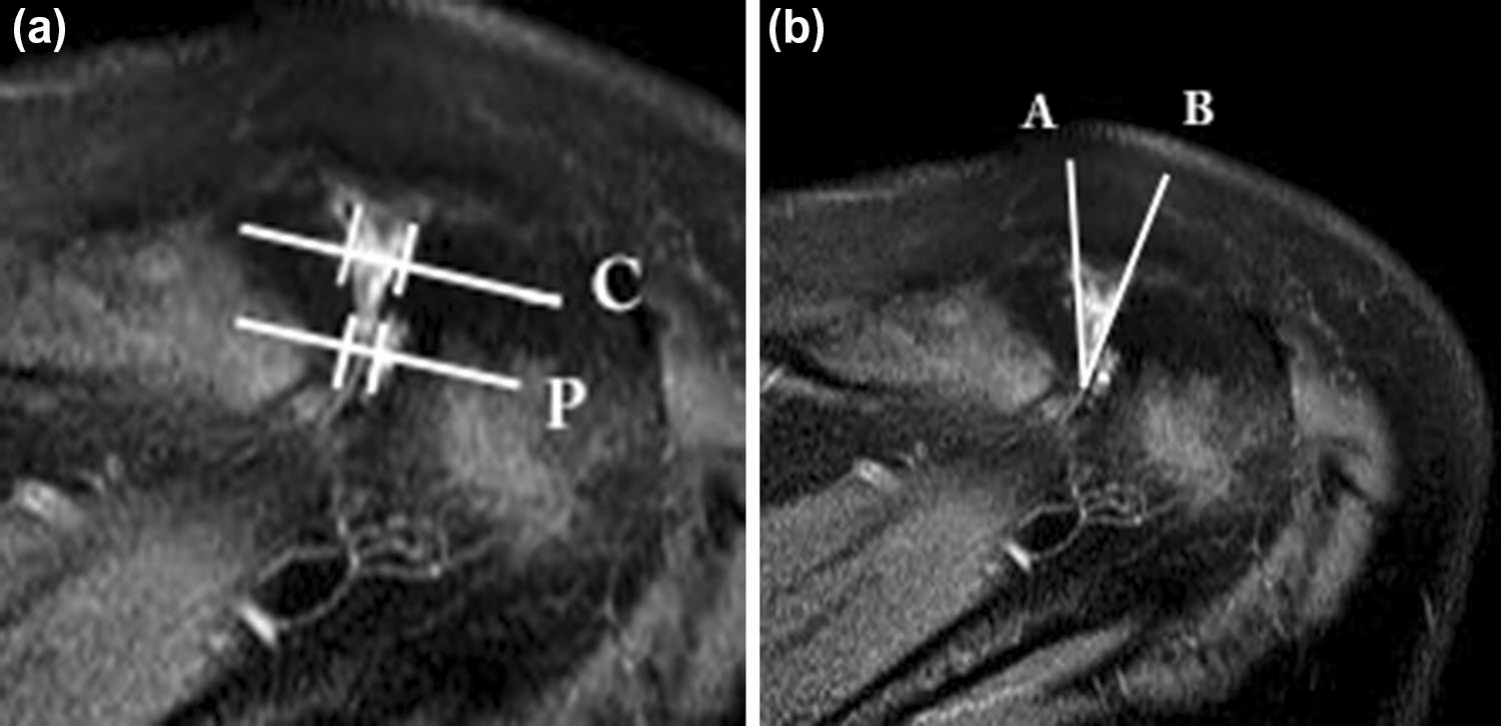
a On MRI scans in the transverse plane with fluid-sensitive sequences (T2-weighted images, T1-weighted images with contrast medium or comparable sequences), the central and posterior joint space width of the AC joints was measured. The distance between both perpendicular bars on line C represents the central joint space width and the distance between both perpendicular bars on line P represents the posterior joint space width. b The AC angle was measured between two lines that are aligned with the joint surface of the acromion (line A) and the lateral clavicle (line B), respectively (an anteriorly open angle defined with positive values)
